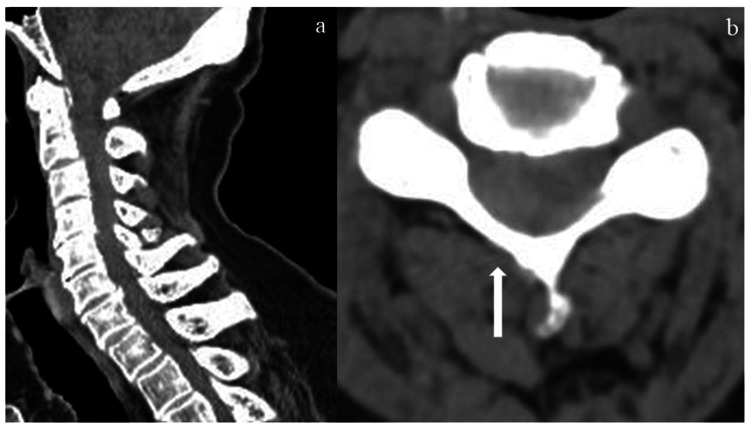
CT scan of cervical spine in a soft tissue window (a) Sagittal CT scan of the cervical spine. (b) Axial CT scan of the cervical spine revealed a right-sided shadow suggestive of an epidural hematoma.
